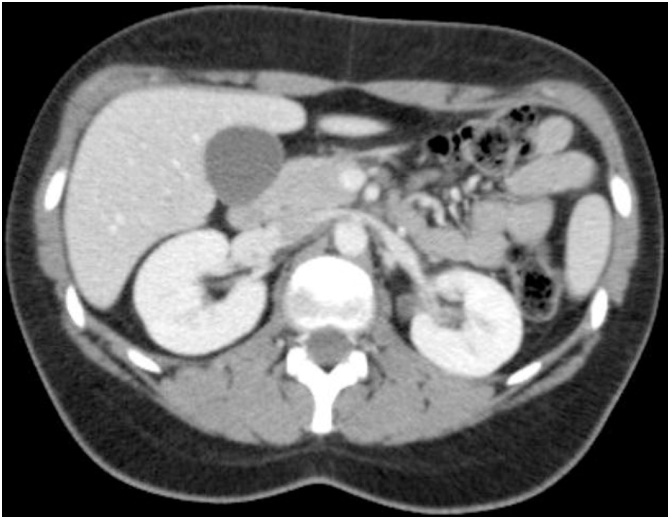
CT scan, axial view, showing absence of hydronephrosis bilateral.
